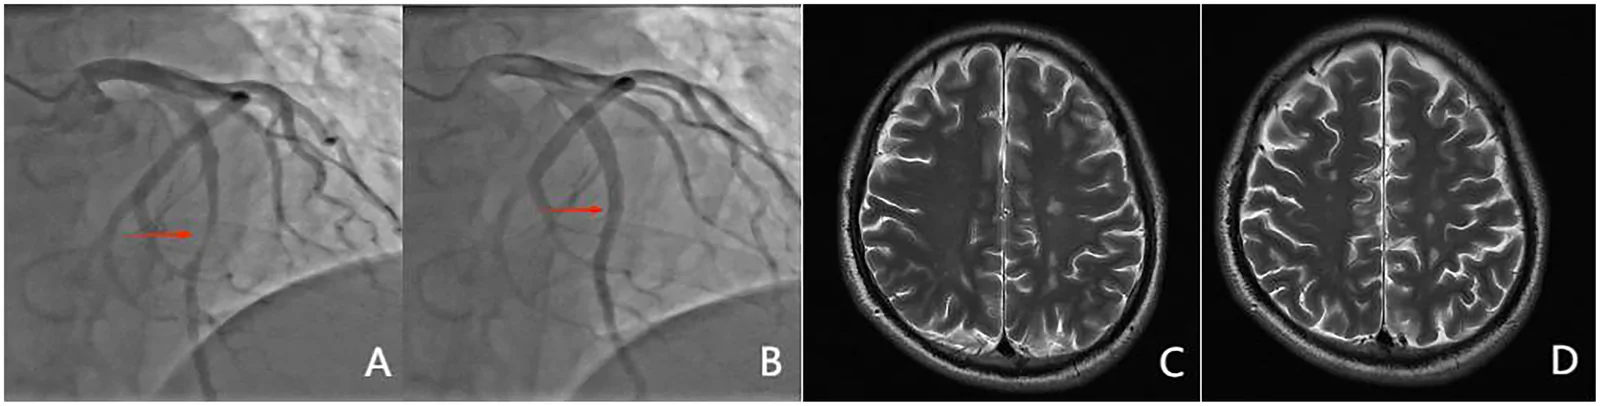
Coronary angiography and brain magnetic resonance imaging. (A) A systolic coronary angiographic frame shows approximately 80% dynamic compression of the mid-left anterior descending artery due to myocardial bridging (red arrow), without a fixed obstructive lesion. (B) Diastolic coronary angiography showed no compression of the mid-portion of the left anterior descending artery (red arrow). (C,D) Axial T2-weighted brain MRI images show multifocal hyperintense infarct lesions in the bilateral frontoparietal regions.
